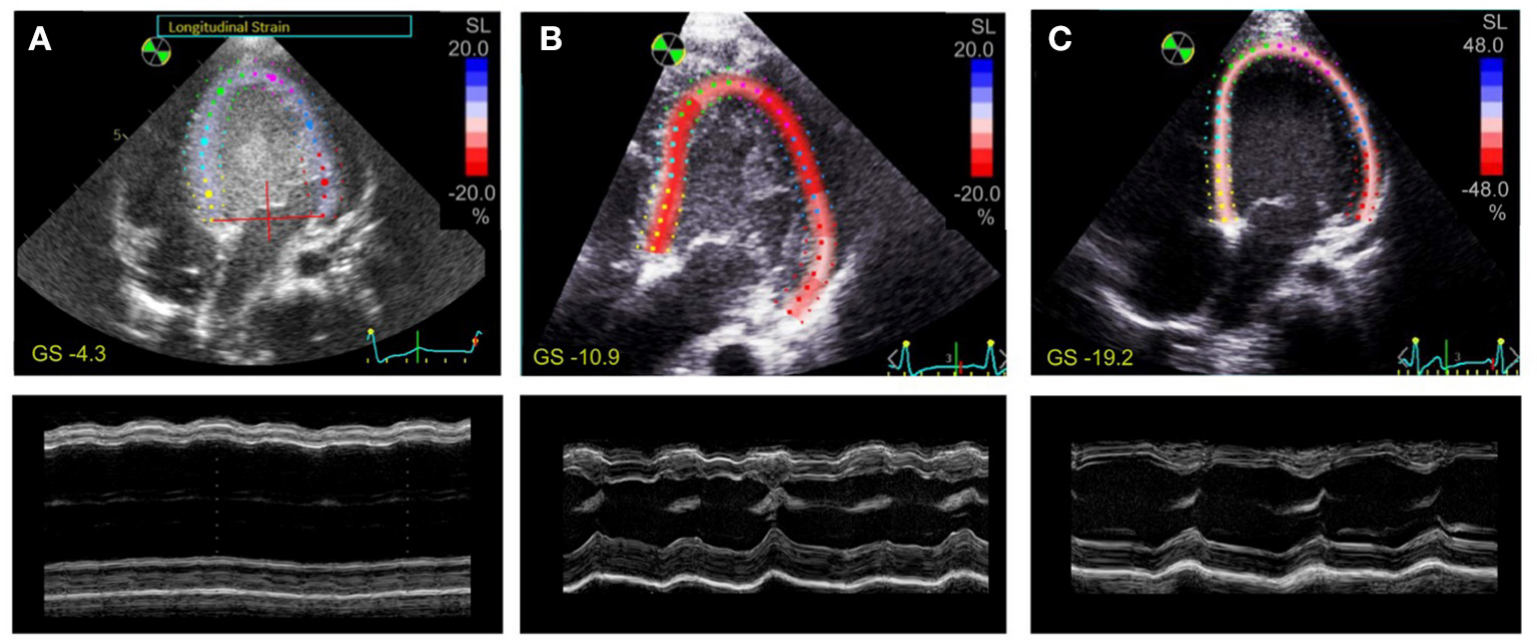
Speckle tracking and M-mode echocardiography of a 21-month old patient with fulminant viral myocarditis. Apical 4-chamber views with tracing of the endo- and epicardium and longitudinal strain (SL) of the left ventricle (*top*), and M-mode view (*bottom*) (A) 1 day before VAD implantation, (B) after 1 month of VAD therapy, (C) after 3 months of VAD therapy. Global strain (GS) is indicated in yellow. Note the different color scaling in (C).
